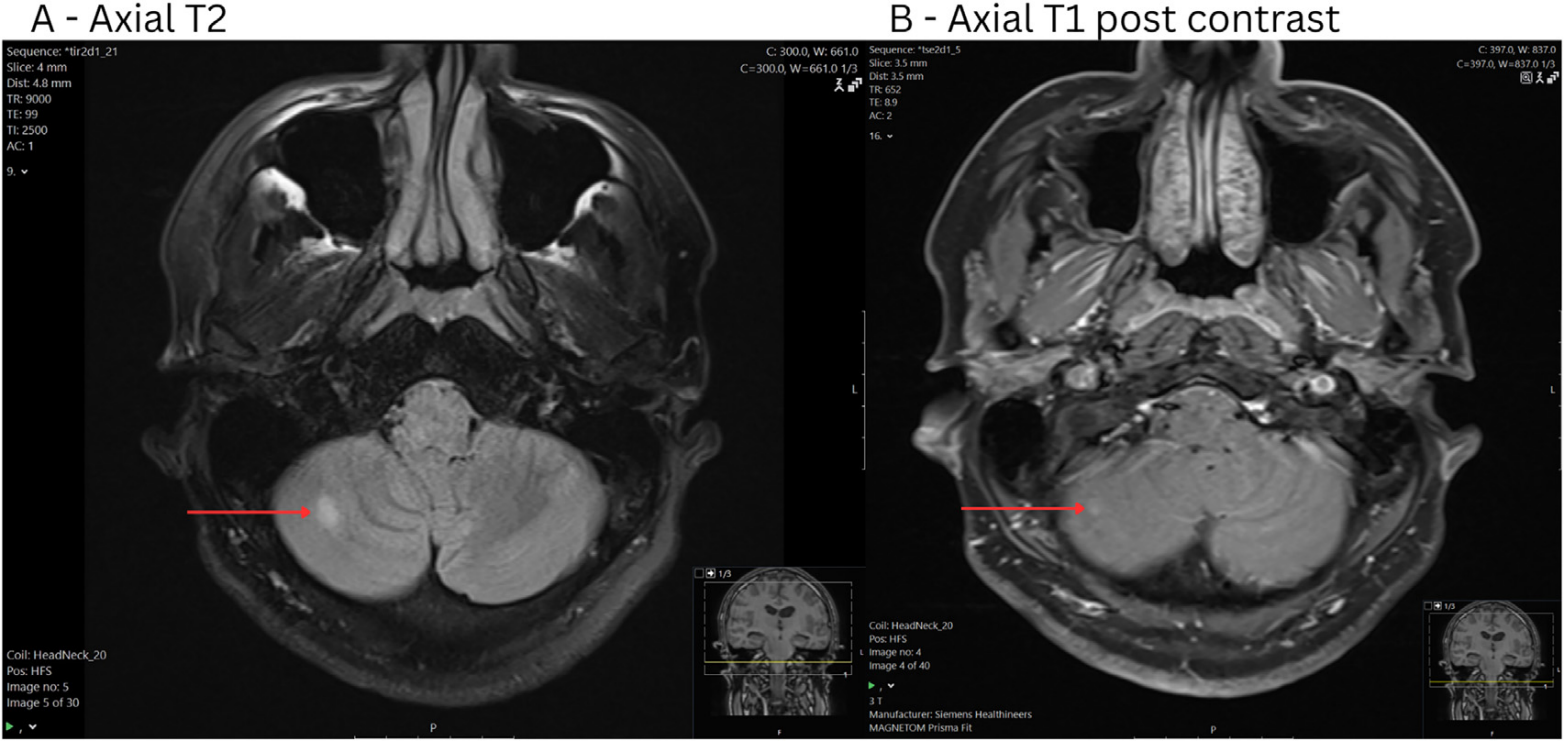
MRI images of a right cerebellar lesion including: (A) Axial T2, (B) Axial T1 post contrast. Total 7 mm subtle enhancing T2/FLAIR hyperintense mass right inferolateral cerebellum. DWI sequence demonstrated diffusion restriction.
